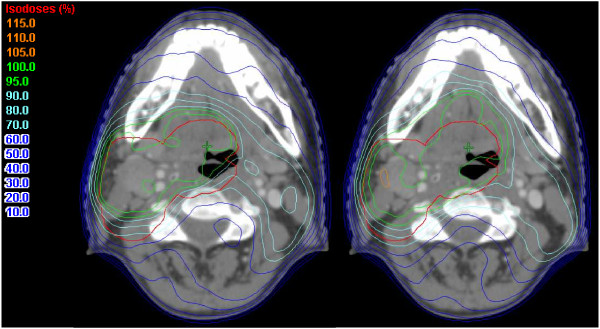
PTV_RT _and the dose distributions optimized for PTV_C _(left) and optimized for PTV_D _(right). PTV_RT_: PTV delineated on the CT registered with the MRI scan in the radiotherapy position; PTV_C_: PTV delineated using the CT with the diagnostic MRI scan viewed on a separate console; PTV_D_: PTV delineated on the CT registered with the diagnostic MRI scan.
